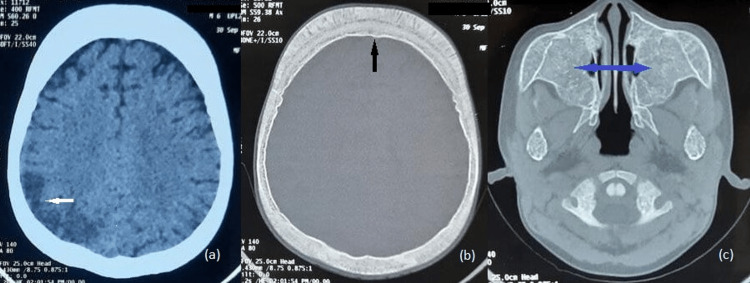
Non-enhanced axial CT brain. (a) Hypodensity in the right parietal cortex with loss of gray-white matter differentiation (white arrow). (b) Axial CT brain in the bone window showing thickened calvarium with widening of diploic space in the frontal bones (black arrow). (c) Absent pneumatization of bilateral maxillary sinuses (blue arrow).
